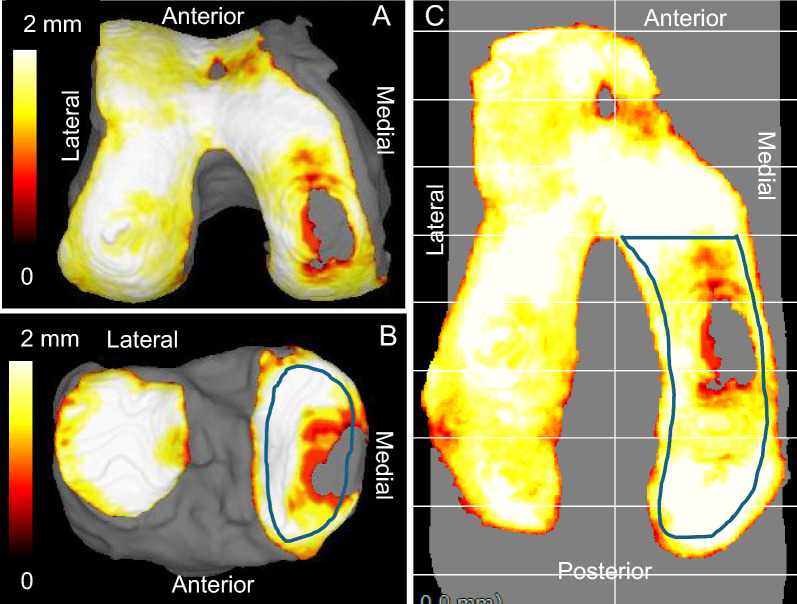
Cartilage thickness mapping and regions of interest for cartilage area ratio. A Vertically projected cartilage thickness mapping of the femoral cartilage generated from three-dimensional (3D) MRI analysis. B Vertically projected cartilage thickness mapping of the tibial cartilage, with the corresponding medial tibial region of interest (ROI). C Radially projected cartilage thickness mapping of the femoral cartilage, with the corresponding medial femoral ROI. Cartilage thickness is displayed using a color scale, with cartilage thickness ≥ 2 mm shown in white. Thinner cartilage is represented by a gradual color change, and areas with complete cartilage loss are shown in gray, indicating exposed subchondral bone. The cartilage area ratio was calculated as the cartilage-covered area divided by the total ROI area
